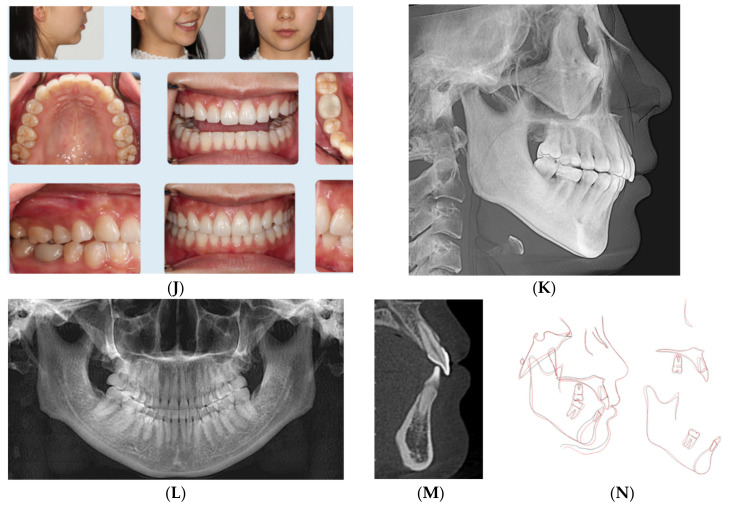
(A) Initial photographs showing the patient’s convex profile with recessive chin and anterior crowding. (B) Initial digital models showing left side Class II buccal occlusion with increased overjet 5 mm. (C) CBCT-driven cephalometric radiograph showing convex profile and recessive chin. (D) CBCT panoramic radiograph showing the patient is missing all third molars; no other noticeable intraoral bony lesion could be detected. (E) CBCT = driven sagittal screen of lower incisor showing its severe proclination with minimum bone appearing on most of the labial surface of the root. (F) A photo of the Jaw Bra (Chanhassen, MN USA). (G) Digital treatment plan (ClinCheck) showing posterior teeth distalization, mesio-bucaal rotation and intrusion to help forward rotation of the mandible to full Class I. (H) Upper arch digital treatment plan (ClinCheck) showing expansion (blue—initial teeth position, white—final teeth position). (I) Lower arch digital treatment plan (ClinCheck) showing expansion (blue—initial teeth position, white—final teeth position) to allow lower incisor intrusion and leveling /alignment of lower incisor crowding. (J) Final photos showing improved patient’s profile and chin projection as well as aligned upper and lower incisors and Class I buccal occlusion both sides. (K) CBCT-driven final cephalometric radiograph showing improved profile and chin projection (L). Final CBCT-driven panoramic radiograph. (M) Final CBCT-driven sagittal screen radiograph of upper and lower incisors showing improved bone labial to both upper and lower incisors. (N) Superimposition of cephalometric tracing of before (black) and after (red) treatment showing improved forward chin projection, palatal root torque of upper incisors, and profile and chin projection and lingual tipping of lower incisors.
